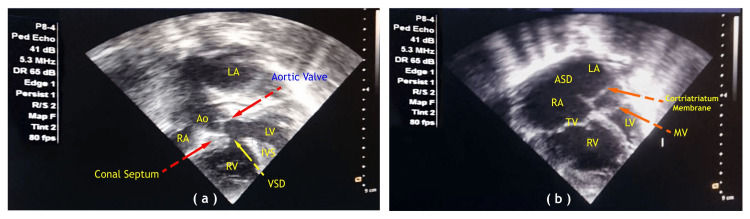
Preoperative 2D echocardiography apical four-chamber view showing (a) ventricular septal defect in case one and (b) cortriatriatum membrane in case two. RA: right atrium; LA: left atrium; ASD: atrial septal defect; AO: aorta; RV: right ventricle; LV: left ventricle; TV: tricuspid valve; MV: mitral valve; IVS: interventricular septum; VSD: ventricular septal defect
